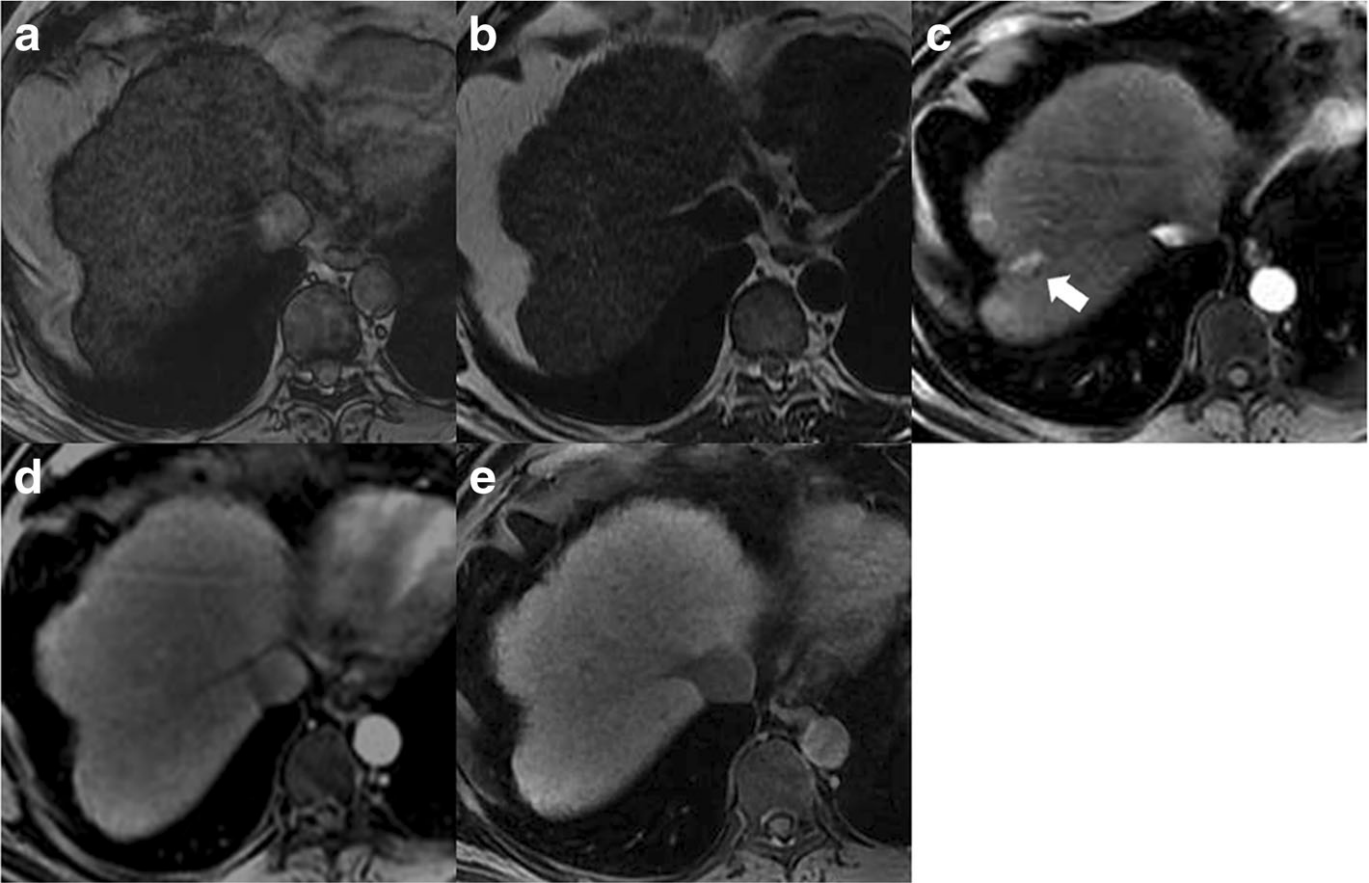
MR scans in a patient with chronic hepatitis, HCV related, and a regenerative nodule in liver segment VIII. a-b T1 - and T2-weighted images do not reveal any focal liver lesion. c T1-weighted gradient-echo shows a hypervascular lesion without wash-out during the late dynamic phase d). On the corresponding MR image obtained during the liver-specific hepatobiliary phase (e), the lesion is isointense to adjacent hepatic parenchyma. At pathologic examination of the explanted liver, this lesion corresponded to a multiacinar cirrhotic nodule
